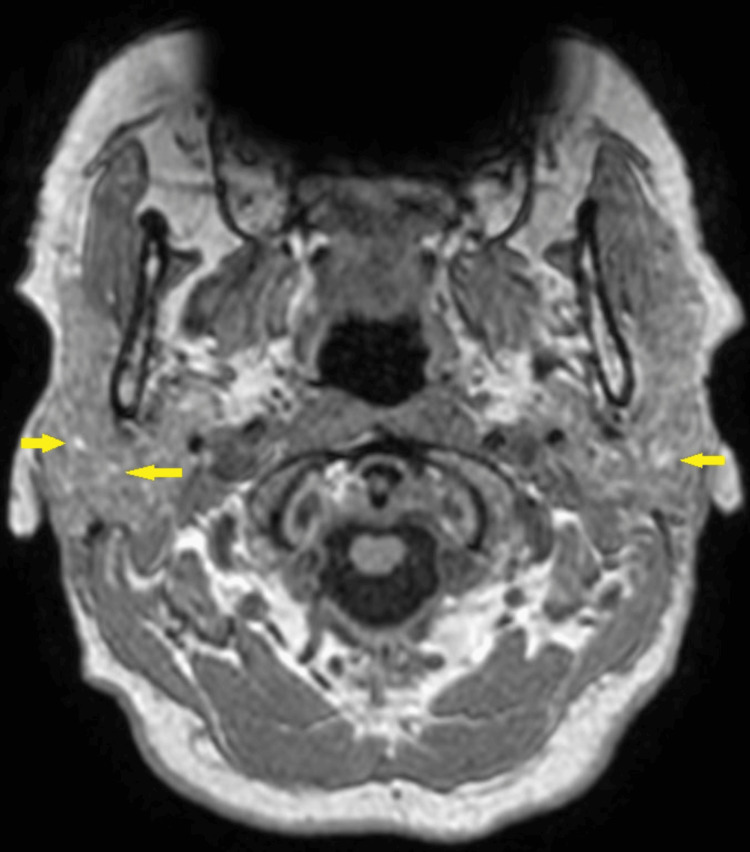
T1-weighted image depicts uneven distribution of speckled fat signal (yellow arrows) in the parotid glands. Staging system for conventional imaging of the parotid glands proposed by Rao et al. [14], grade 1.
